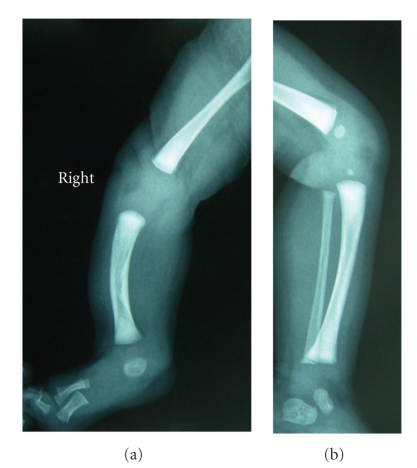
Radiograph showing absence of right fibula and shortening and bowing of right tibia at the 7th postnatal day.
